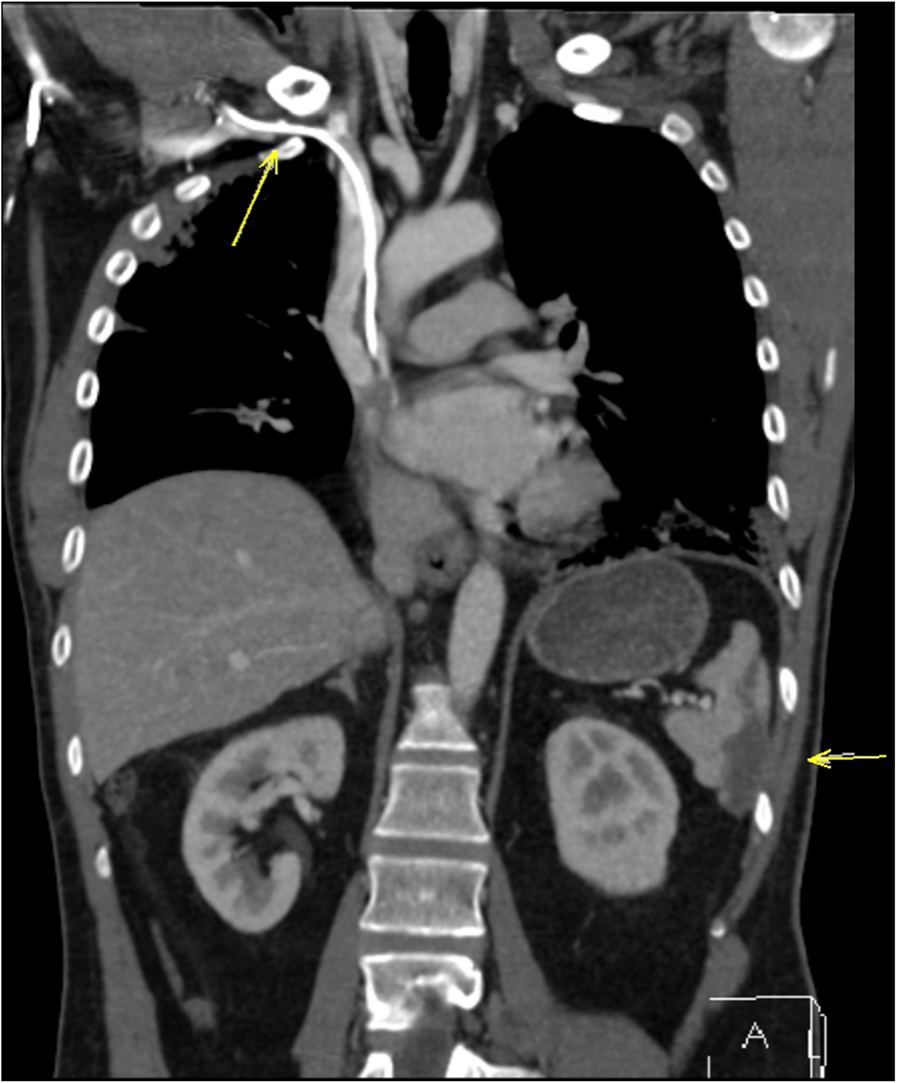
Computed tomography of chest and abdomen, coronary section, performed after third cycle of chemotherapy, showing the central venous port device with thrombosis (arrow) and subtotal splenic infarction (arrow).
